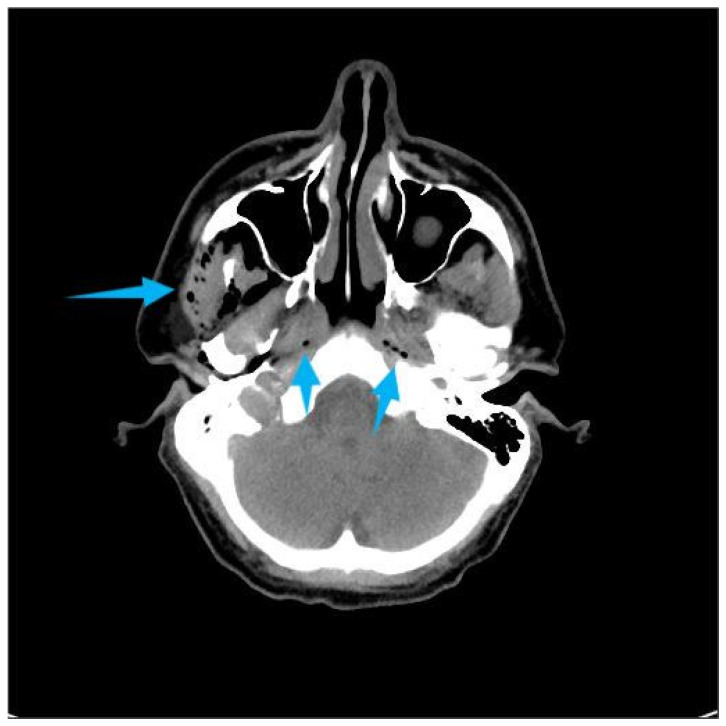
Axial Multislice Computed Tomography (MSCT) image showing a submucosal emphysema of the pharynx and a subcutaneous emphysema of the right side of the face. The arrows highlight the presence of emphysema.
